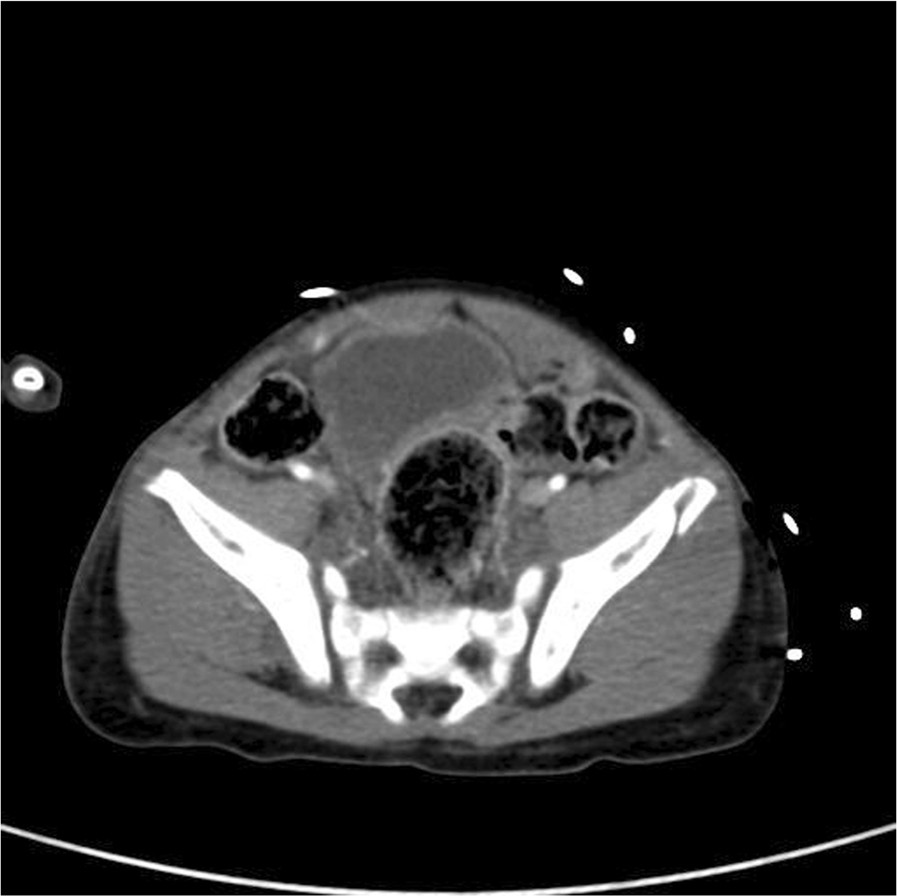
Initial contrast-enhanced axial CT scan. The scan shows multiple fractures of the pelvic bone and the hematoma formed in the paravesical and prevesical retroperitoneum.
